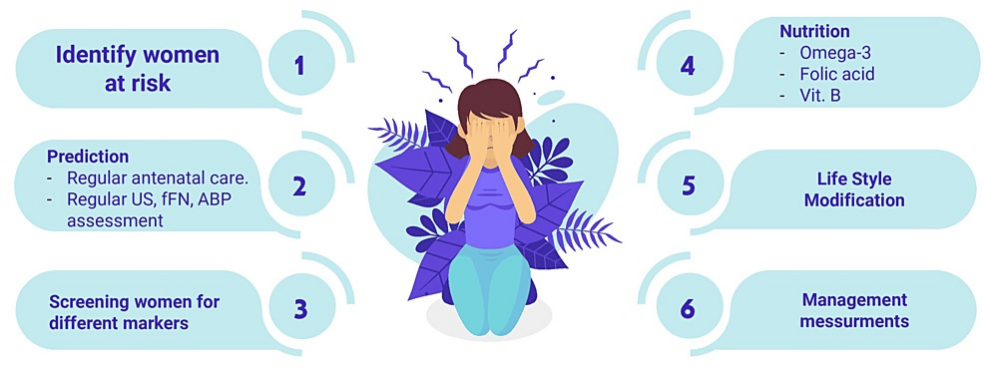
Prevention of preterm birth in high-risk women ABP: arterial blood pressure; fFN: fetal fibronectin; US: ultrasound; Vit.: vitamin Image Credit: Authors
